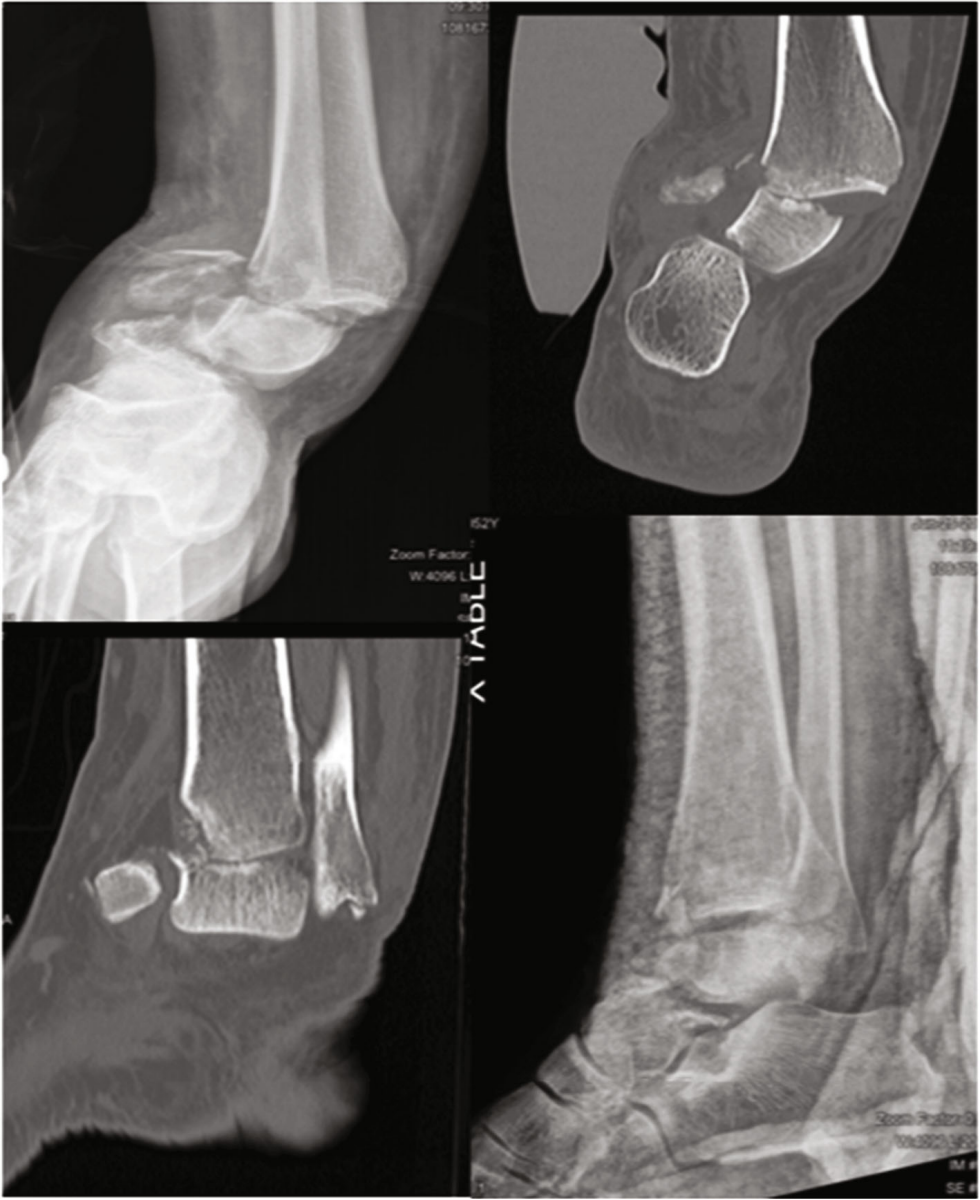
Injury films and CT scan demonstrated 180° inversion of proximal talus such that the dome was adjacent to the subtalar joint. The talus remained inverted after initial closed reduction attempt in the emergency department.
